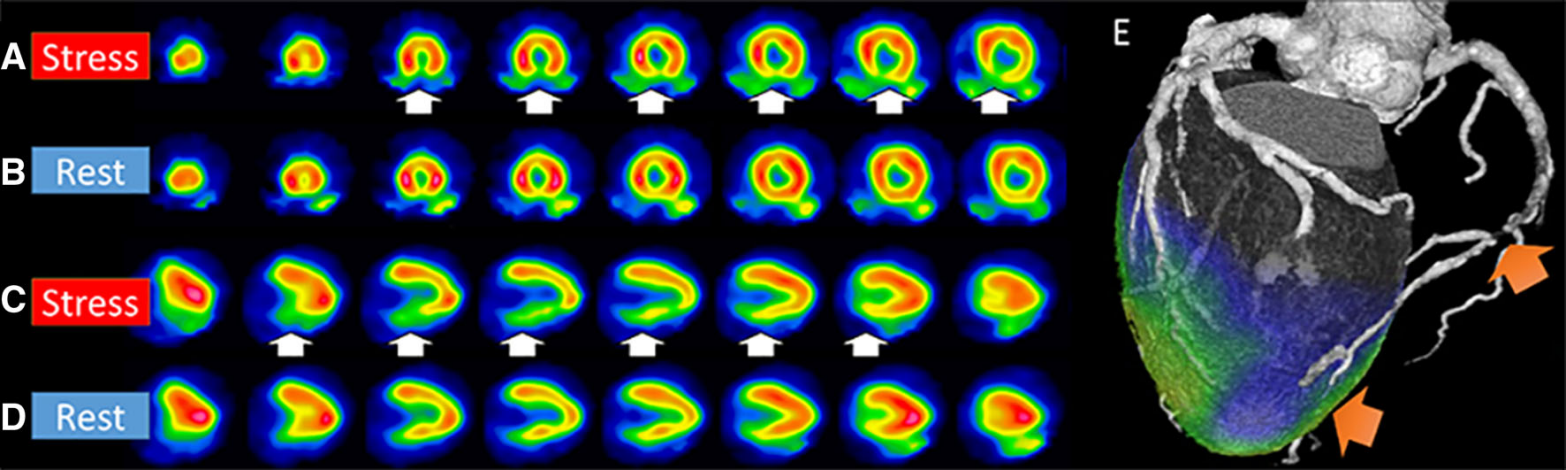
Myocardial perfusion images Perfusion images of short-axis image at stress (A) and rest (B), vertical long-axis image at stress (C) and rest (D) using ^99m^Tc-product, and fused image of stress perfusion and CT coronary angiography (CTCA; E) are displayed. Severe perfusion reduction is detected in the inferior wall at stress (white arrows). Fill-in is seen at rest indicating stress-induced ischemia in the right coronary artery (RCA). CTCA revealed significant stenosis in the RCA (orange arrows)
